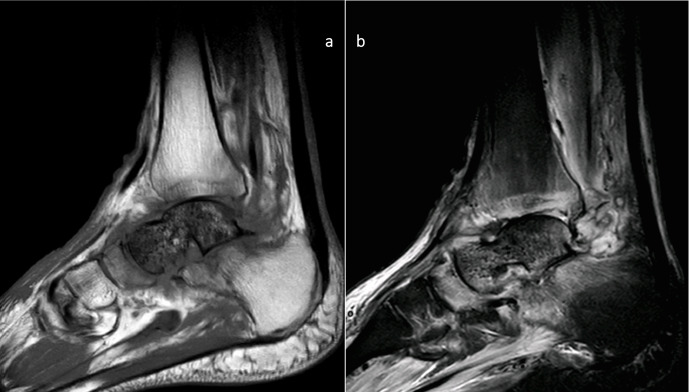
MRI images of the preoperative state of the talus. Sagittal MRI images showing signs of avascular necrosis and osteomyelitis: (a) T1 and (b) STIR images.
